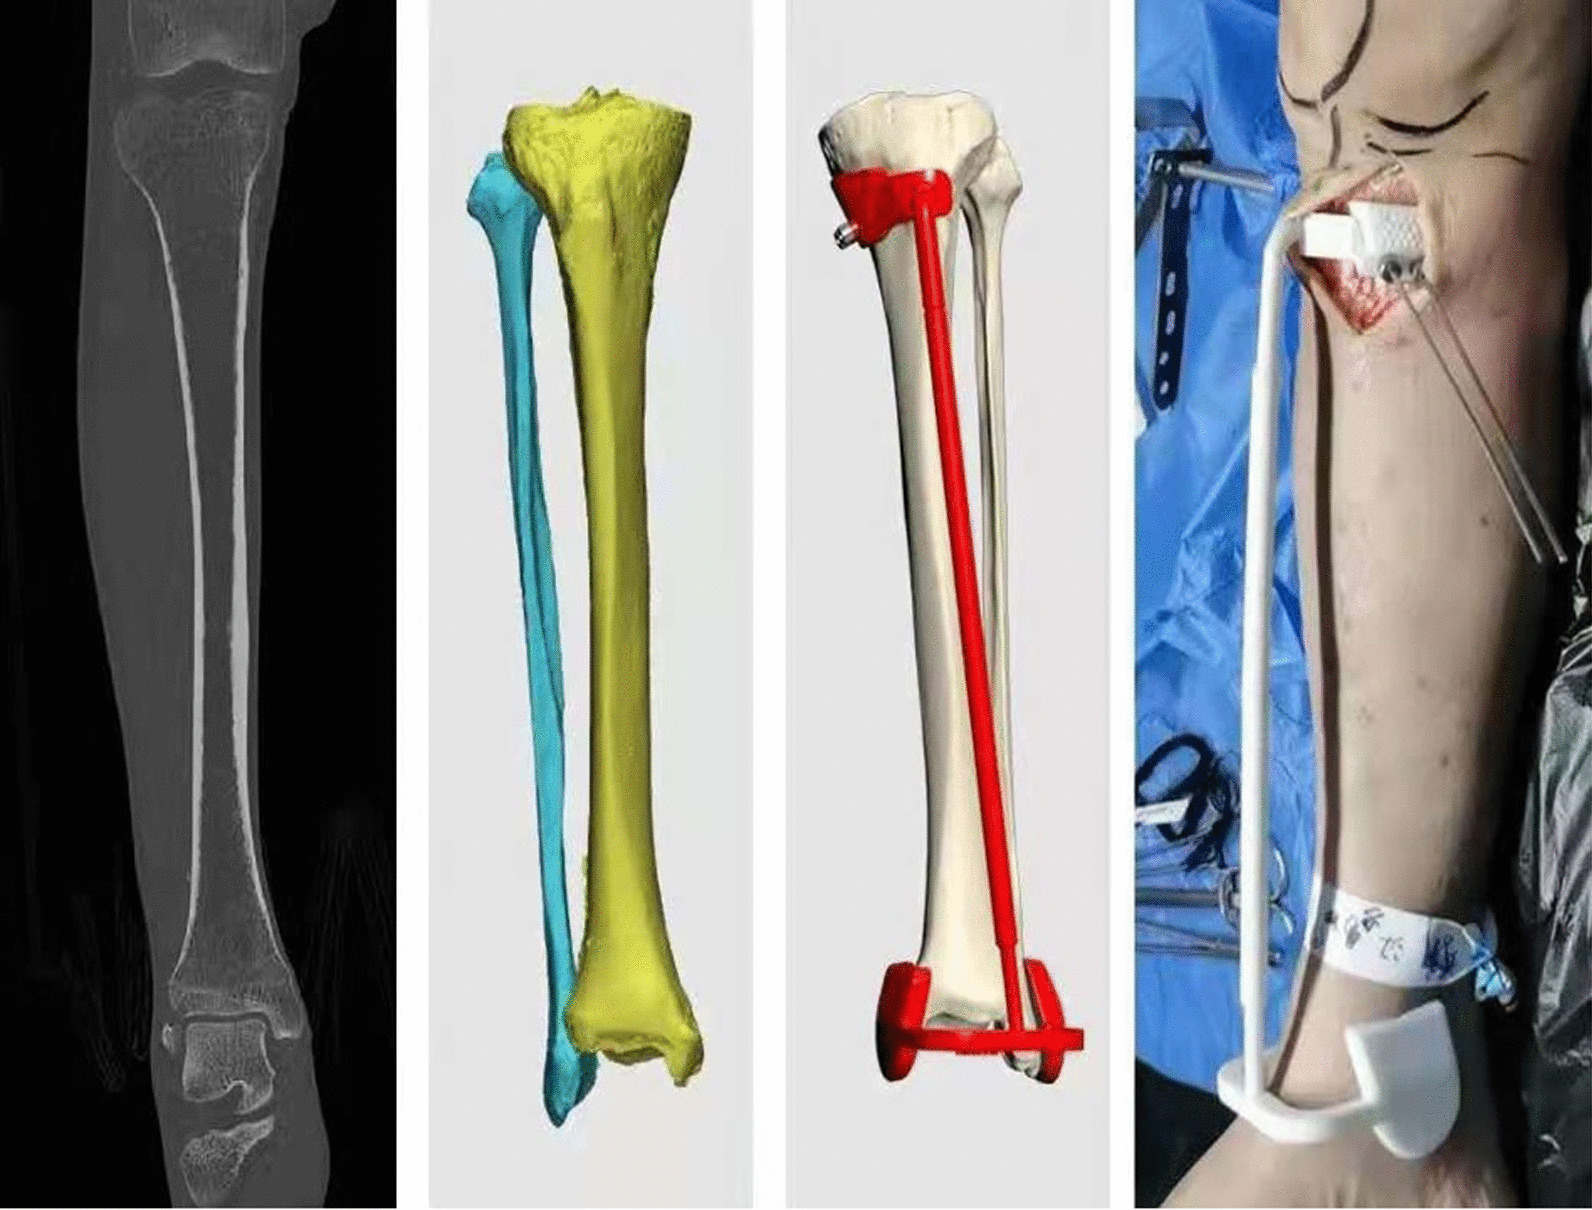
Design and 3D printing of PSI according to the preoperative plan and 3D skeletal model of the lower extremities (a perform CT examination. b CT scans were imported into Mimics 25.0 software to construct a 3D skeletal model of the lower limb. c The PSI was designed based on the preoperative plan and the 3D skeletal model of the lower extremities. d The PSI was 3D-printed)
